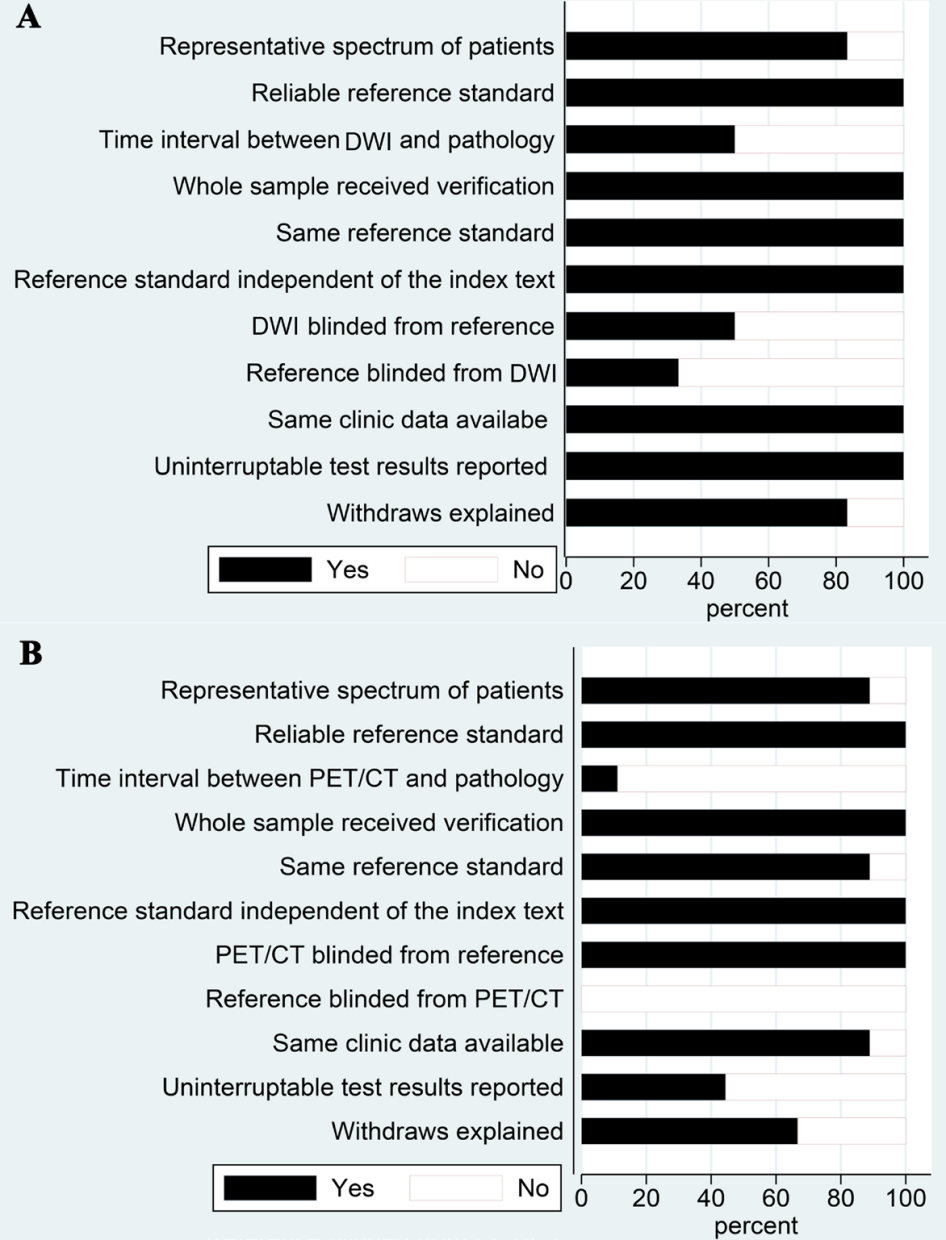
Quality assessment of included studies using QUADAS-2 (A) For DWI studies; (B) For ^18^F-FDG PET/CT studies.
